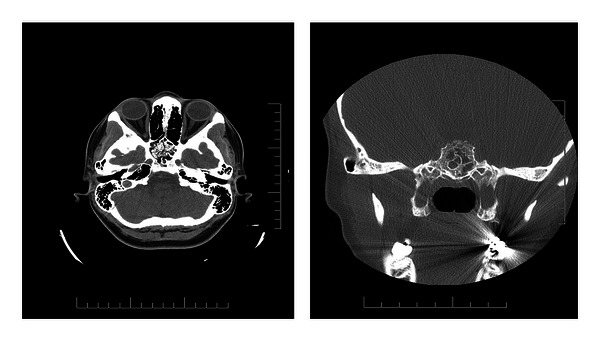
Routine CT scan revealed intraosseous lipoma within the sphenoid bone and sinus with small intratumor calcifications.
